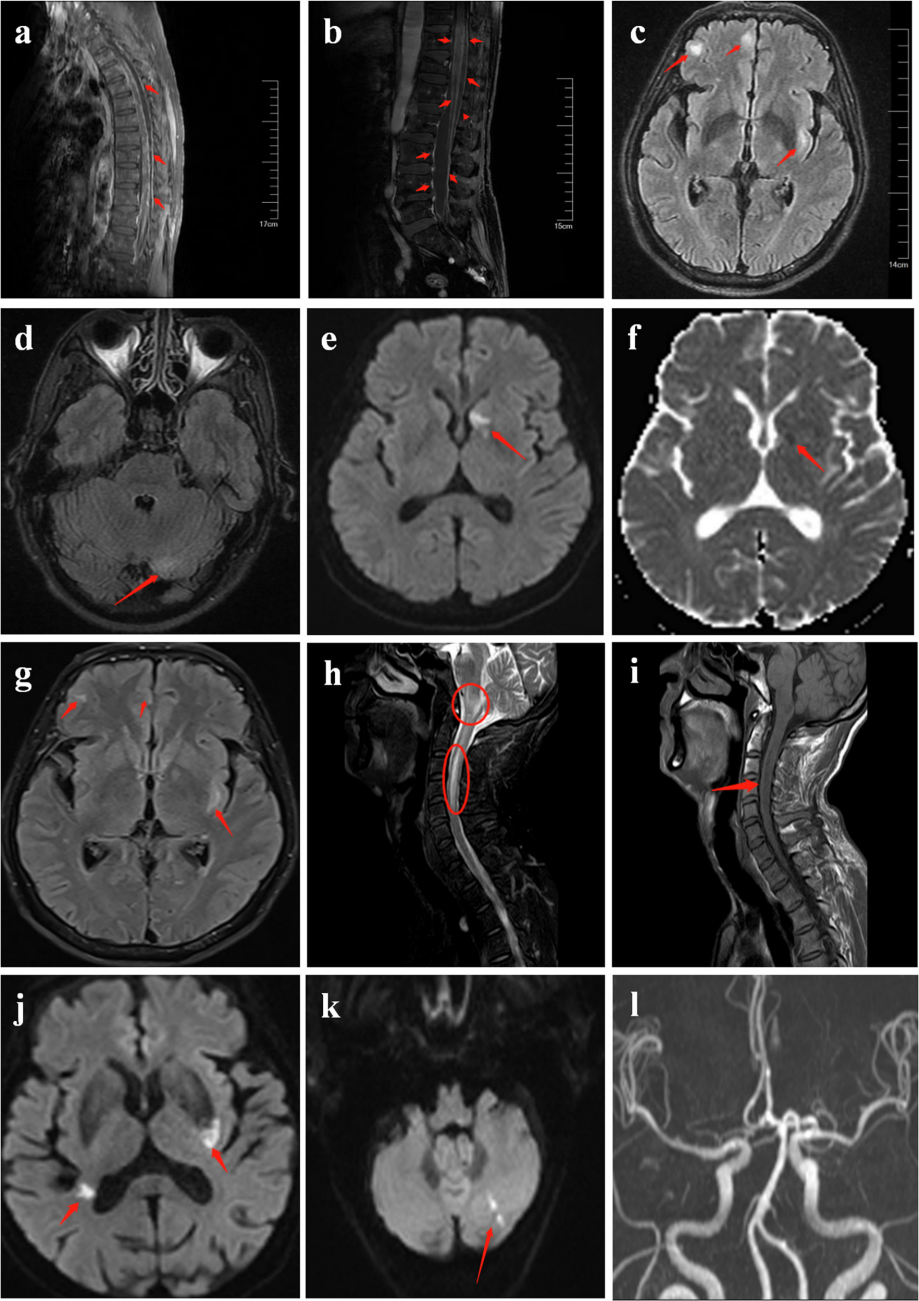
Neuroimaging data of the patients. (a and b) Thoracic and lumbar spine MRI of case 1 showing diffuse irregular thickening of the myelomeningocele. (c and d) Brain MRI of case 9 showing bilateral frontotemporal, parietal, left insula, and multiple nodular in the bilateral cerebellum hemispheres. (e–g) Brain MRI of case 9 showing new patchy foci in the left basal ganglia were appreciated or acute infarction. (h and i) Brain and cranial MRI of case 14 showing abnormal signal of the pontine, medulla oblongata, and cervical spinal cord at the C2–C5 levels. (j–l) Brain MRI of case 15 revealing acute infarction of cerebellar hemispheres, frontal lobe, basal ganglia, corona radiata, the right basal ganglia, and multiple spot shadows in the lateral ventricles.
